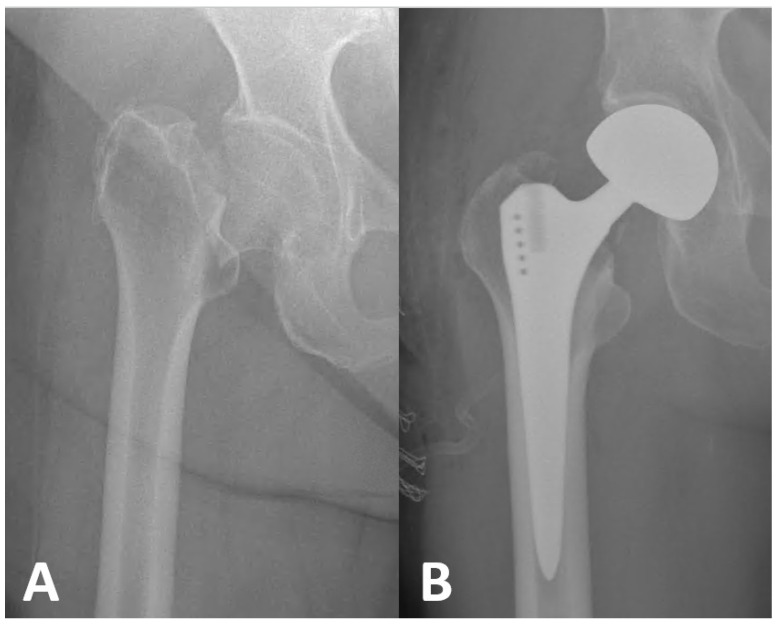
(A) Pre-operative anteroposterior radiograph of femoral neck fracture in a 72-year-old geriatric patient. (B) Post-operative anteroposterior radiographic view of this patient who underwent hip bipolar hemiarthroplasty (size 46 mm head and size 5 femoral stem) using a cementless femoral stem.
